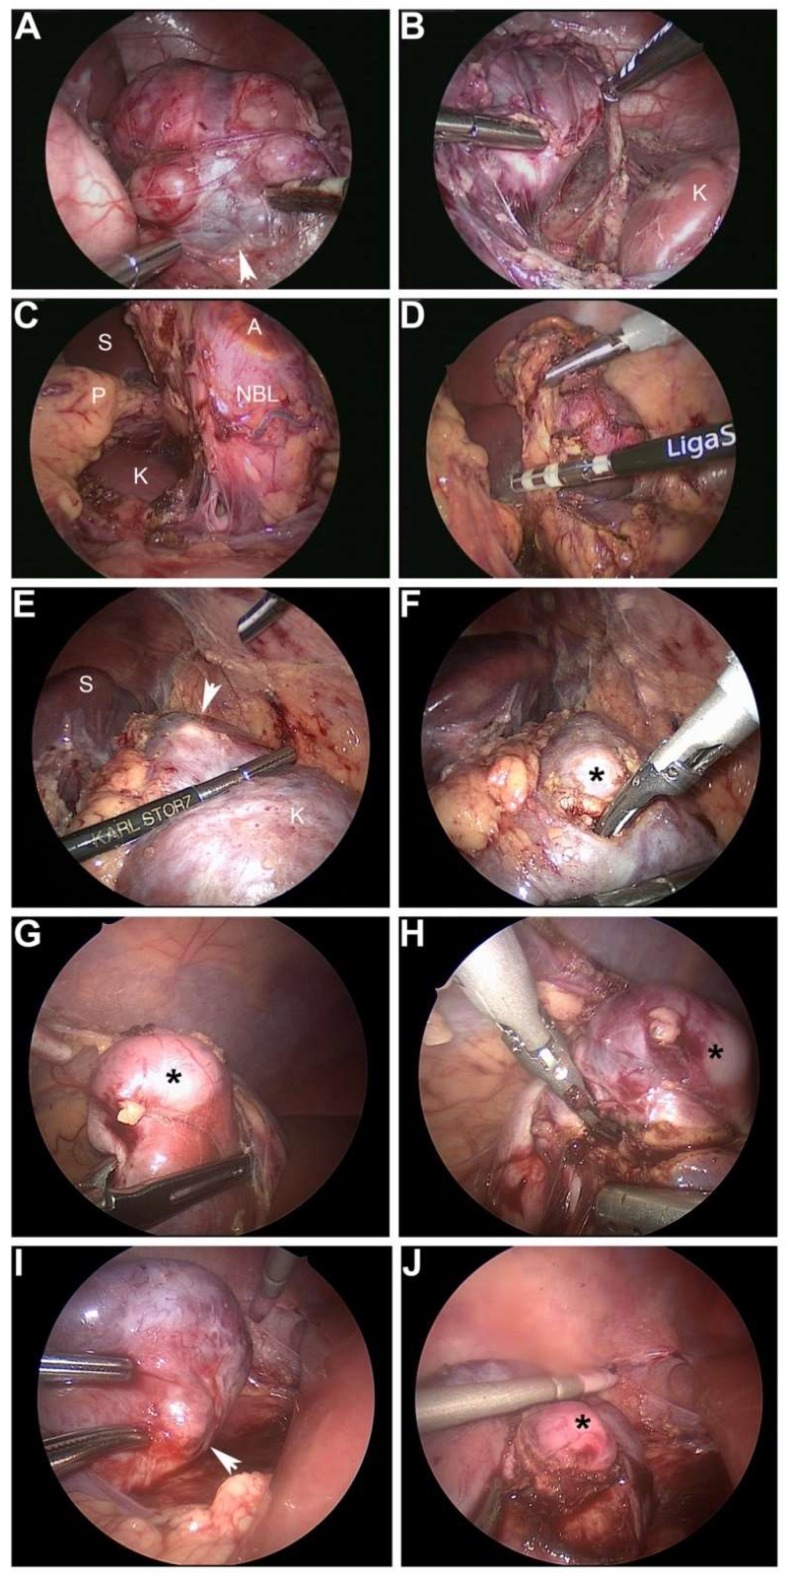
MIS resection of neuroblastoma and Wilms tumor. (A,B) 20-month-old male who was diagnosed with a left adrenal neuroblastoma secreting vasoactive intestinal polypeptide. Arrowhead shows confluence of adrenal vein (draped across mass) with left renal vein (A). (B) Dissection of NBL away from left kidney (labeled K). (C,D) Infant who presented with opsoclonus-myoclonus and was discovered to have a left adrenal NBL (labeled; A, adrenal; S, spleen; P, pancreas; K, kidney). Complete resection with negative margins was achieved in both cases using a bipolar energy vessel sealer. (E,F) 18-month-old male with Beckwith–Wiedemann syndrome who was discovered on routine cancer screening to have a left renal mass consistent with Wilms tumor. Images show resection with ultrasonic scalpel of residual mass in left upper pole after six weeks of neoadjuvant therapy. Arrowhead and asterisk denote 1 cm mass (S, spleen). (G–J) Four-year-old girl who had been treated in infancy for diffuse hyperplastic perilobar nephrogenic rests. On routine cancer screening, two right renal cortical masses consistent with Wilms tumor were discovered. (G,H) MIS resection of lower pole Wilms tumor (asterisk) with ultrasonic scalpel. (I,J) Resection of right upper pole Wilms tumor, also with ultrasonic scalpel (arrowhead and asterisk).
